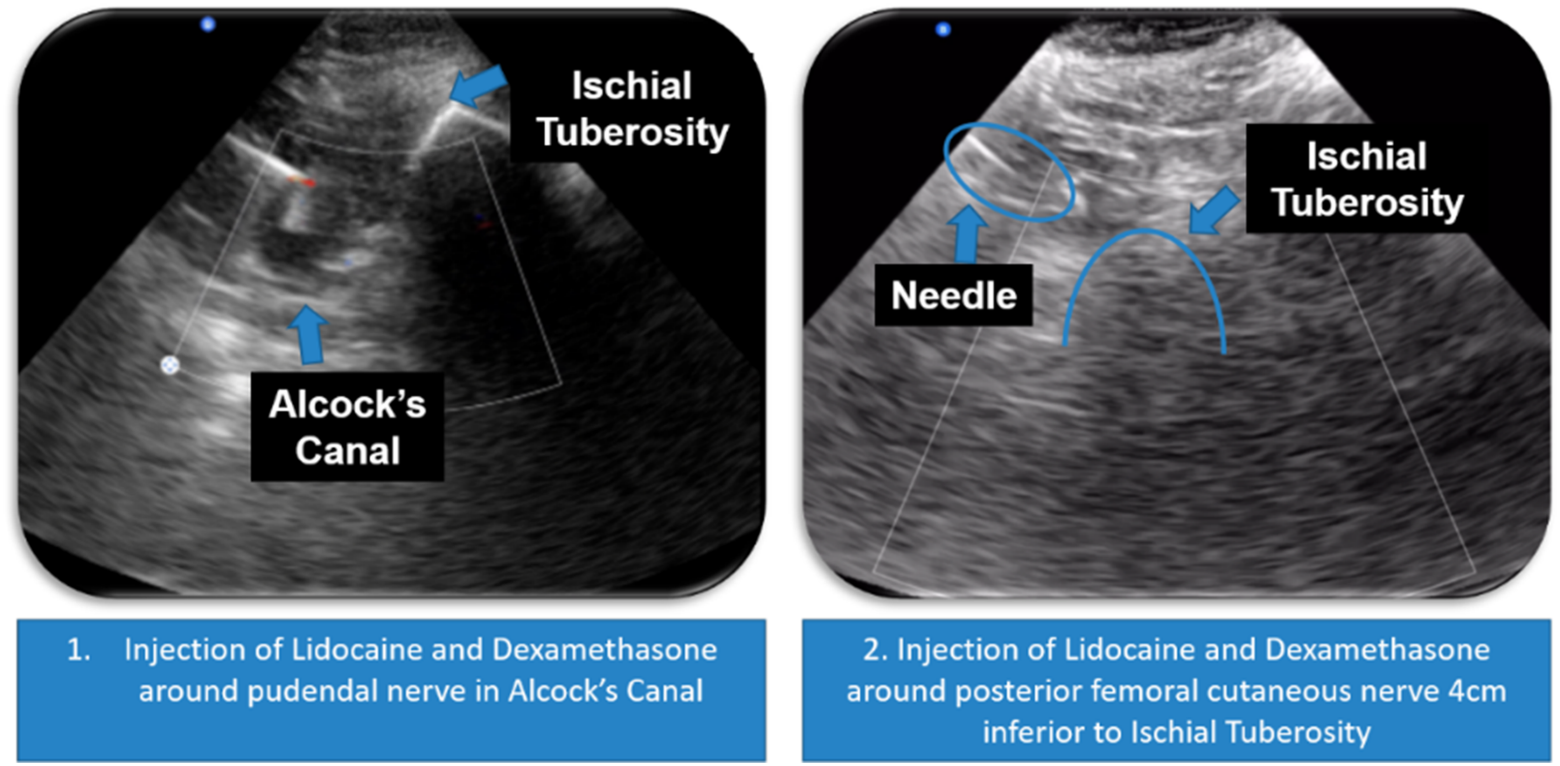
Ultrasound images of Alcocks Canal and Obturator Canal
